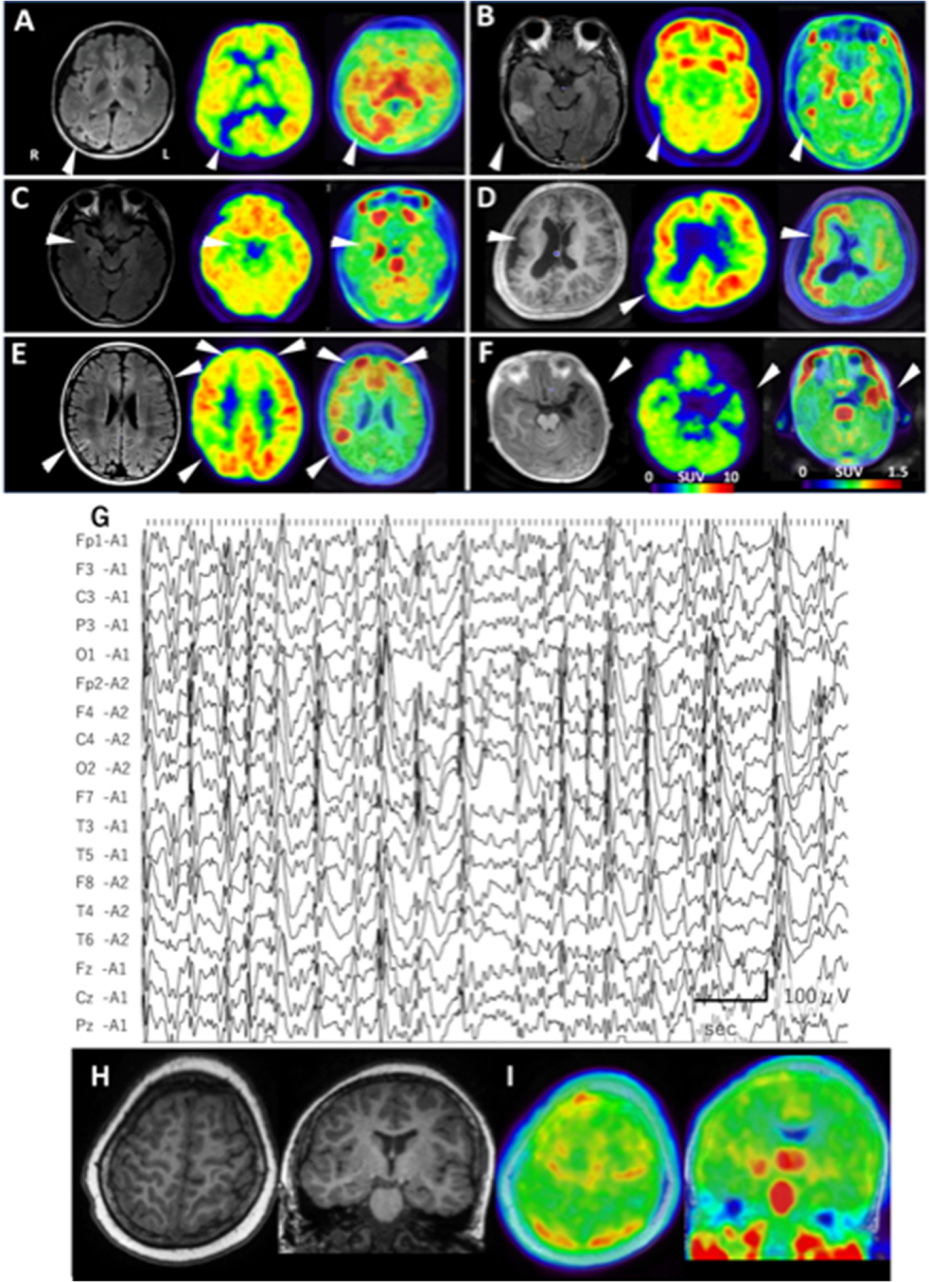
Neuroimaging data of six selected patients (a–f). Left, MRI; middle, FDG-PET; right, [^11^C] DPA713PET. a Patient 2. MRI showed ulegyria in the right occipital cortex. In this area, hypometabolism, as indicated by FDG-PET, and increased [^11^C] DPA713 uptake, as indicated by TSPO PET, were observed. b Patient 3 had low-grade gliomas with a high-intensity area in the middle temporal cortex, as indicated by FLAIR-MRI. Increased [^11^C] DPA713 uptake on TSPO PET was clearly visible compared with hypometabolism on FDG-PET. C, In patient 5, the right hippocampus showed subtle atrophy, but no obvious high intensity, on FLAIR-MRI and hypometabolism on FDG-PET. In addition, the right hippocampus showed clearly increased [^11^C] DPA713 uptake on TSPO PET. d Patient 9 with 1p36 deletion syndrome showed broad cortical malformation in the right hemisphere on T1-MRI. Although FDG-PET showed hypometabolism in the right temporo-occipital area and hypermetabolism in the right insula-inferior frontal area, TSPO PET demonstrated clearly increased [^11^C] DPA713 uptake in the right hemispheric cortex. e Patient 10 with TSC showed high intensity in the left frontal and right parietal cortex on FLAIR-MRI, suggesting cortical tubers. FDG-PET showed patchy hypometabolism, but the increased uptake area of [^11^C] DPA713 was easy to determine using TSPO PET. F, Patient 15 had atrophy caused by herpes encephalitis in the left temporal cortex and hippocampus on MRI. Although FDG-PET showed broad hypometabolism not only in the left temporal cortex but also in the right temporal cortex, TSPO PET found that the increased uptake area of [^11^C] DPA713 was restricted in the left temporal cortex. Findings obtained from patient 19, who had Landau-Kleffner syndrome (g–i). EEG showed a continuous spike-wave pattern, and the bilateral-centro-parieto-temporal discharges were predominantly observed in the right hemisphere (g). MRI showed no obvious abnormality (h), and TSPO PET revealed increased [^11^C] DPA713 uptake in the bilateral postcentral gyri and right hippocampus (i)
